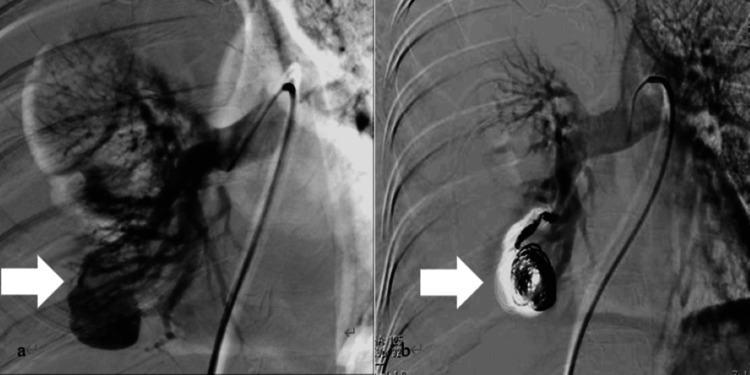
Angiographic findings before and after transcatheter embolization (a) The radiograph shows a pulmonary arteriovenous malformation arising from the right branch of the middle lobar artery. A large aneurysmal sac measuring 25 × 30 mm, with no evidence of intrathoracic leakage of the contrast agent, is visible (arrow). The findings were interpreted as massive hemothorax and tamponade hemostasis due to hematoma. (b) After transcatheter embolization, the early venous return has disappeared, and hemostasis is confirmed (arrow).
